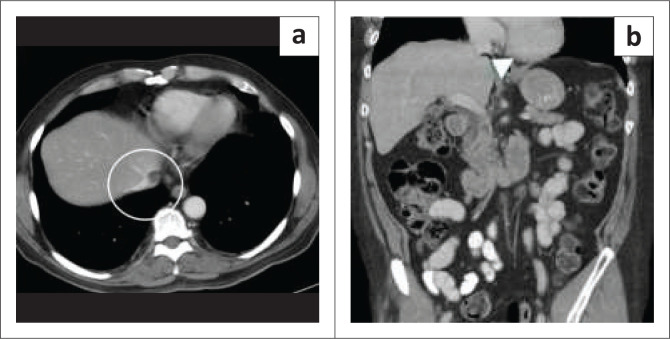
A 47-year-old male evaluated for abdominal pain. The axial (a) and coronal (b) contrast-enhanced CT images demonstrated juxtacaval fat (arrowhead) mimicking an inferior vena cava tumour or thrombus (circle).
